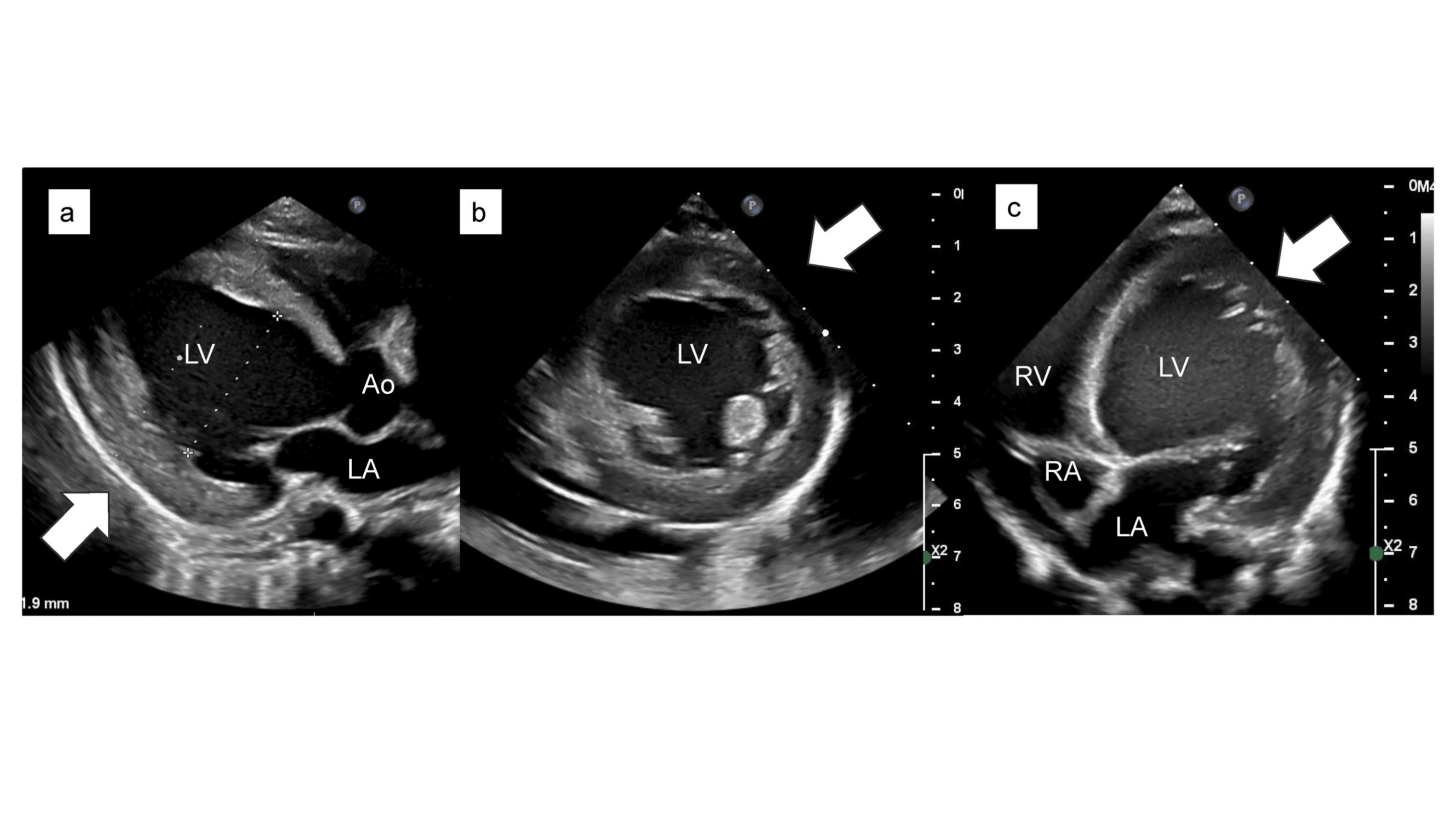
Transthoracic echocardiography at day four after hospitalization. (a–c) Echocardiography in the left ventricular (LV, white arrowhead) (a) long-axis, (b) short-axis, and (c) apical four-chamber views reveal LV enlargement with further reduced ejection fraction (EF) compared to those observed at admission. New-onset LV hypertrophy is observed. RV, right ventricle; RA, right atrium; LA, left atrium; Ao, aorta
